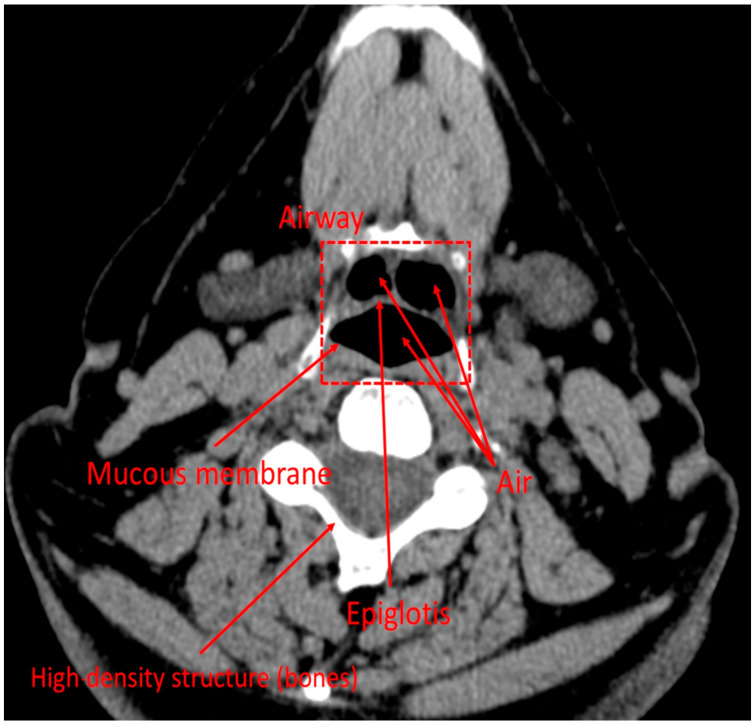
CT image of the supraglottic region in the axial plane.
